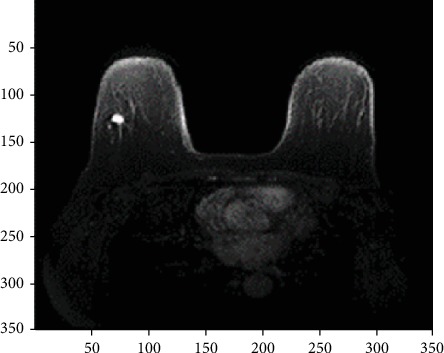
Enhanced sequence MR image of IDC.
